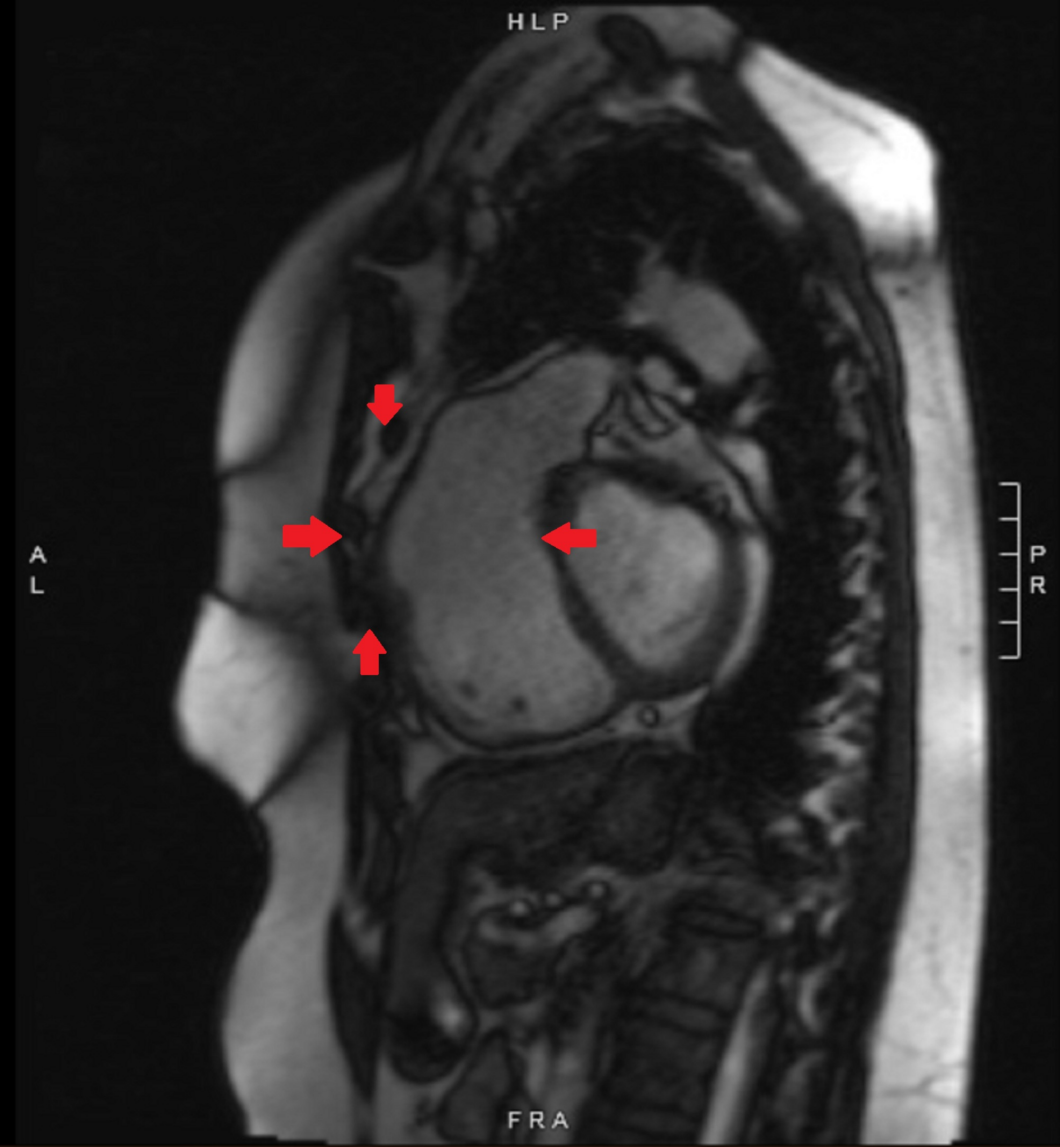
Preoperative cardiac magnetic resonance imaging showing proximity of the heart to the chest wall
